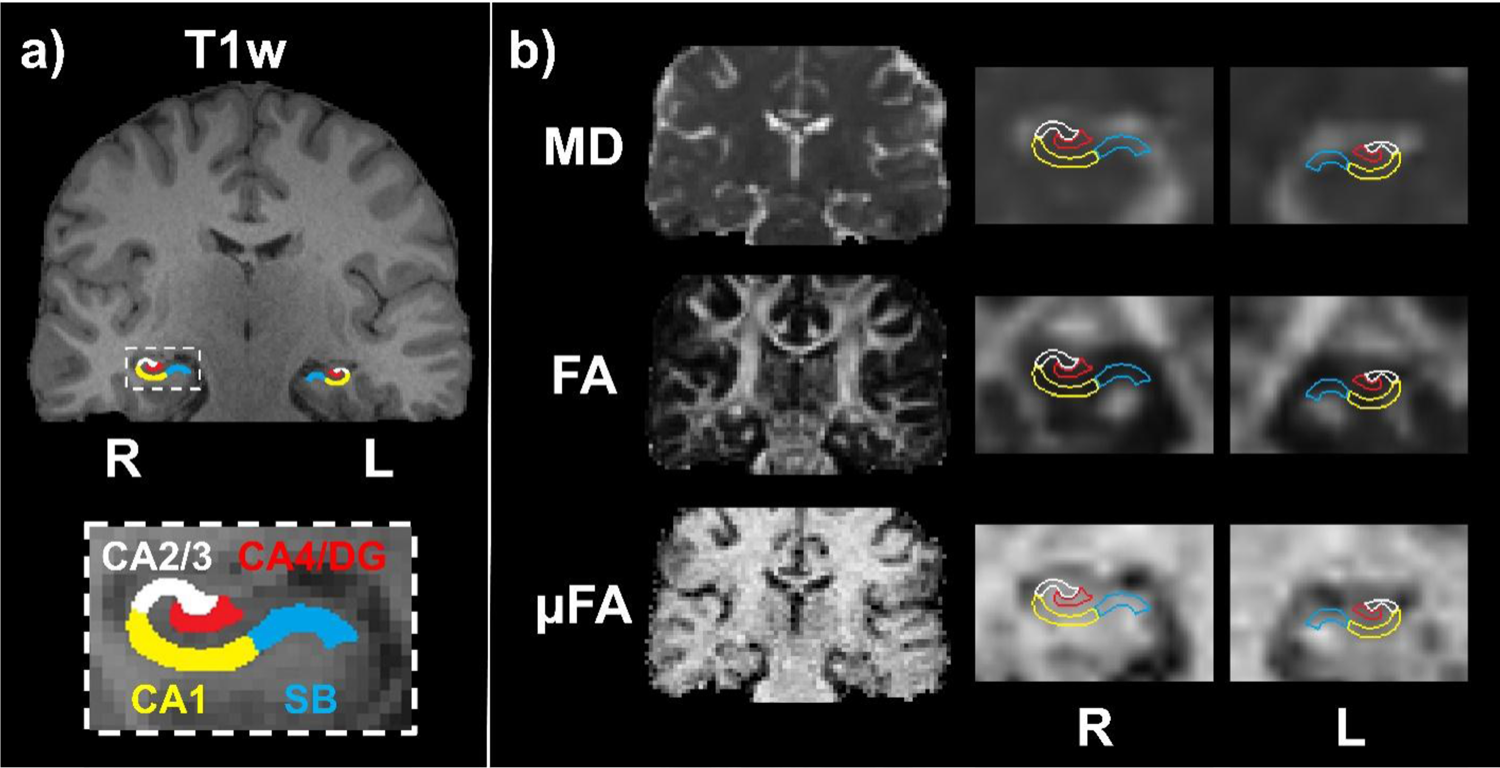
(a) Example T1-weighted coronal image from a drug-resistant TLE patient with unilateral (left) HS evident on structural MRI, with the four hippocampal subregions highlighted. (b) MD, FA, and μFA coronal slices from the same patient before registration to T1-space (left), and after registration to T1-space and interpolation (right) depicting the hippocampal subregions ipsilateral (L) and contralateral (R) to suspected pathology.
